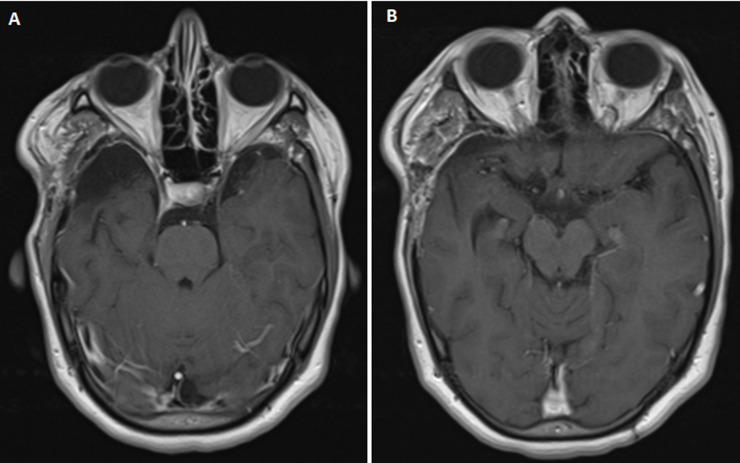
(A,B) two-year follow-up magnetic resonance imaging of the brain: no recurrence
